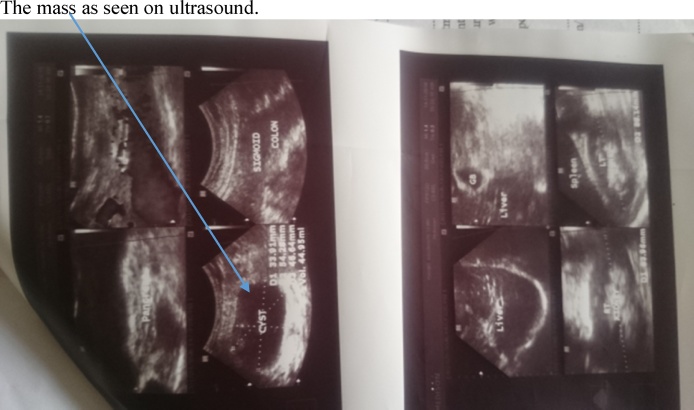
Ultrasound showing the mass appearing cystic.
